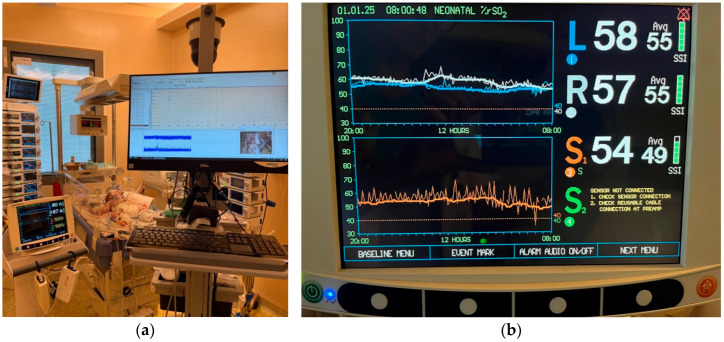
Neuromonitoring of infants following cardiac surgery in the NICU, consisting of (a) amplitude integrated electroencephalography (aEEG) monitoring before the surgery and in the first 24 h postoperative, (a,b) continuous NIRS monitoring perioperative (initiated before the intervention and continued until extubation), as well as transfontanellar Doppler before and after intervention (measuring blood flow velocities and resistivity index).
